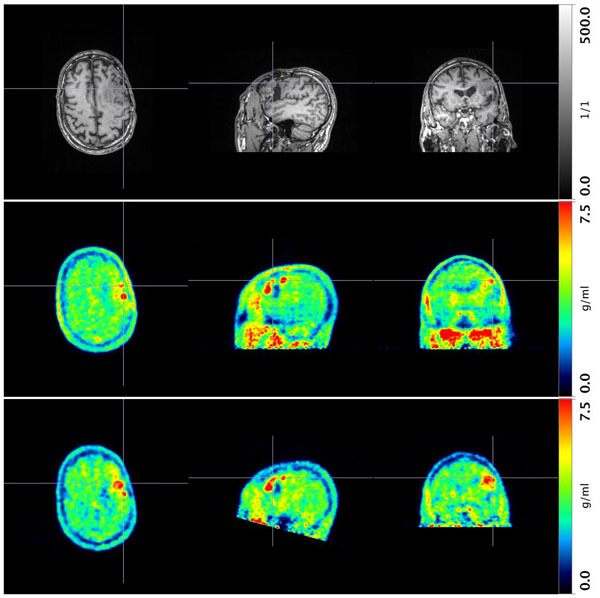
MRI and FET-PET scan of a patient with oligodendroglioma WHO grade II. T1-weighted MR image (MPRAGE) acquired simultaneously to the early PET scan with the 3T MR-BrainPET (top). Summation scan 20-40 min post-injection (BrainPET, middle), summation scan 70-90 min post-injection (HR+, bottom). Volume of FET-positive region (frontal) increased from 3.8ml to 10.7ml.
